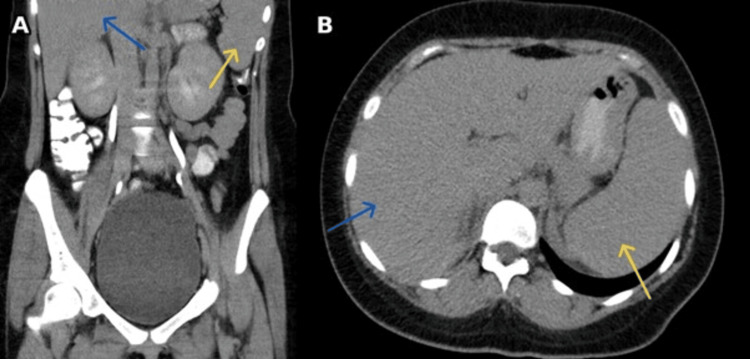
CT of the abdomen and pelvis with contrast Coronal section (A) and axial section (B) showing mild hepatosplenomegaly. The liver is indicated by blue arrows and the spleen is indicated by yellow arrows.
